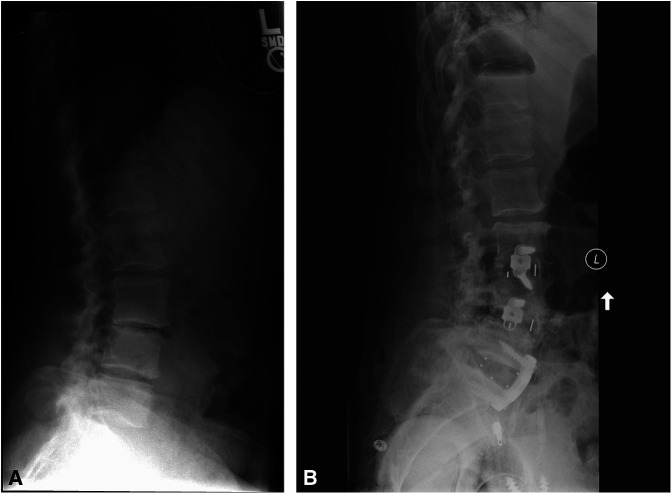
Lateral radiographs of the spine before the spinal fusion (A) and after a three-level spinal fusion conducted from L3 to the sacrum (B).
